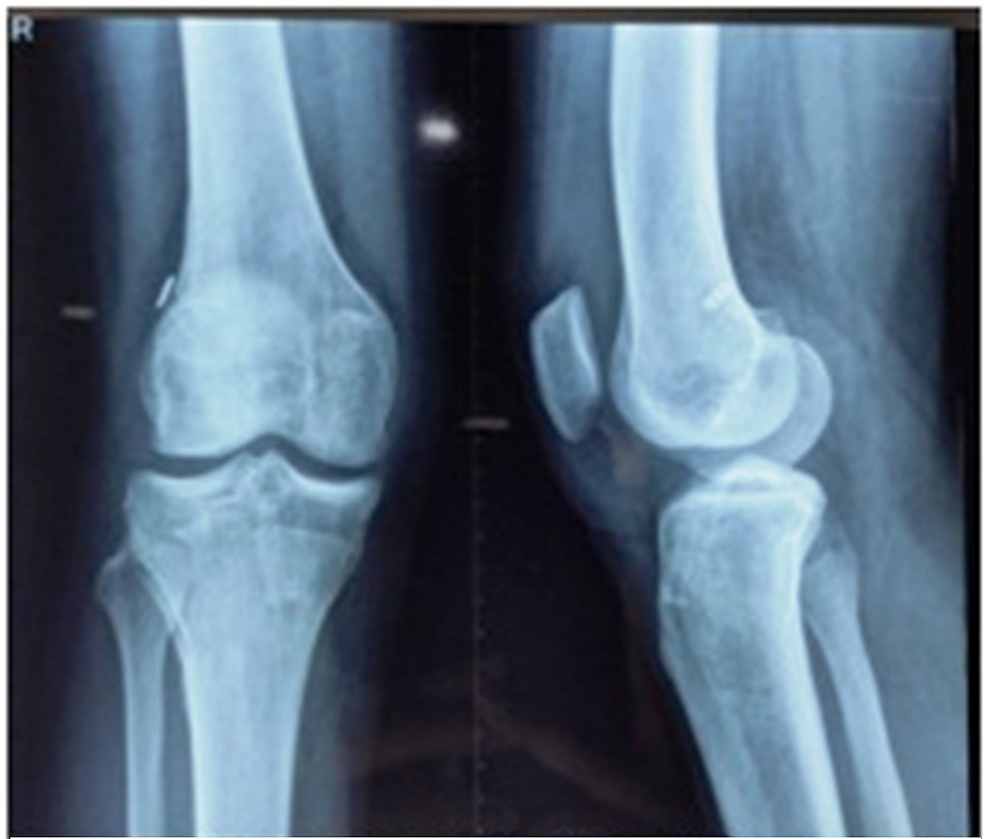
Postoperative radiograph with Endobutton
